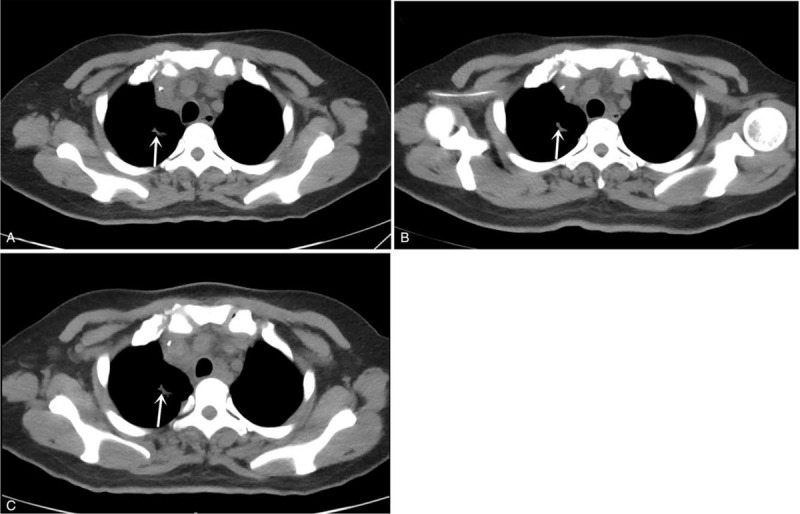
Chest CTs. A-C, Mediastinal window of the chest CT performed on April 01, 2019, showing a mass measuring 10.4 × 4.1 mm in the upper right lung. B, Mediastinal window of the chest CT performed on May 15, 2019, showing a mass measuring 10.3 × 4.0 mm in the upper right lung. C, Mediastinal window of chest CT performed on June 19, 2019, showing a mass measuring 10.3 × 4.0 mm occupying the upper right pulmonary space.
